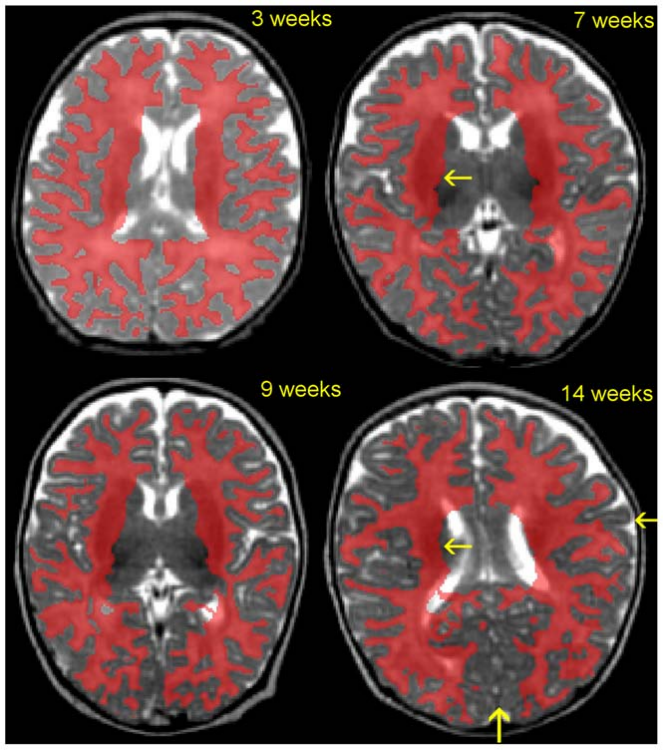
White matter segmentation across age. Segmentations are shown *in red* over axial MRI slices. Despite highly folded patterns, segmentation is accurate at the cortical border for younger infants (3, 7 and 9 weeks); however, segmentation at the subcortical boundary needs improvement (*yellow arrow*). As for infants older than two months old (14 weeks), segmentation performance decreases in a few areas because of on-going maturation, such as along the calcarine sulcus and the central sulcus (*yellow arrows*).
